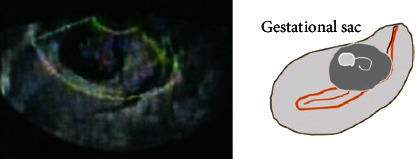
Ultrasound findings at 9 weeks' gestation at a previous clinic. The fetal sac was located in the lower part of the anterior wall of the uterus.
